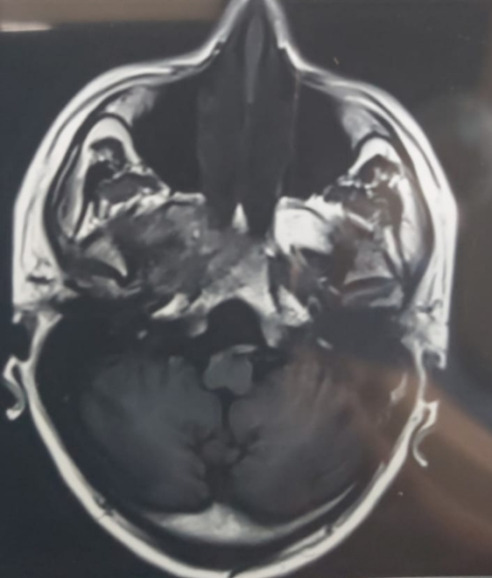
Axial MRI image showing extension into infratemporal fossa and periantral extension
